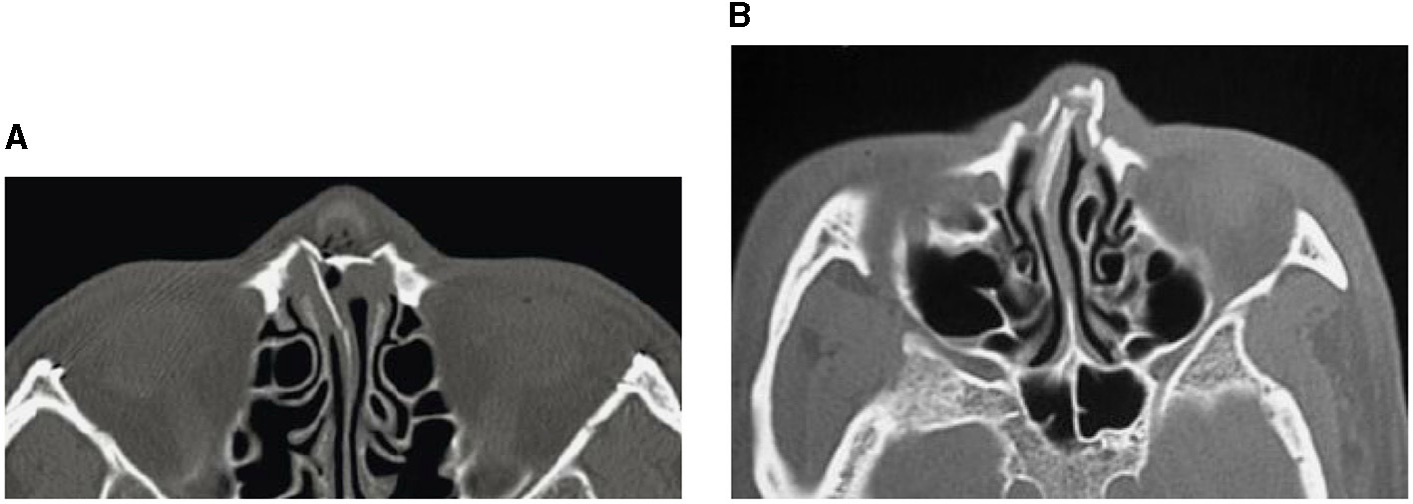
CT images showing nasal bone fractures: across the complete nasal bone (A), and with leftward deviation (B).
